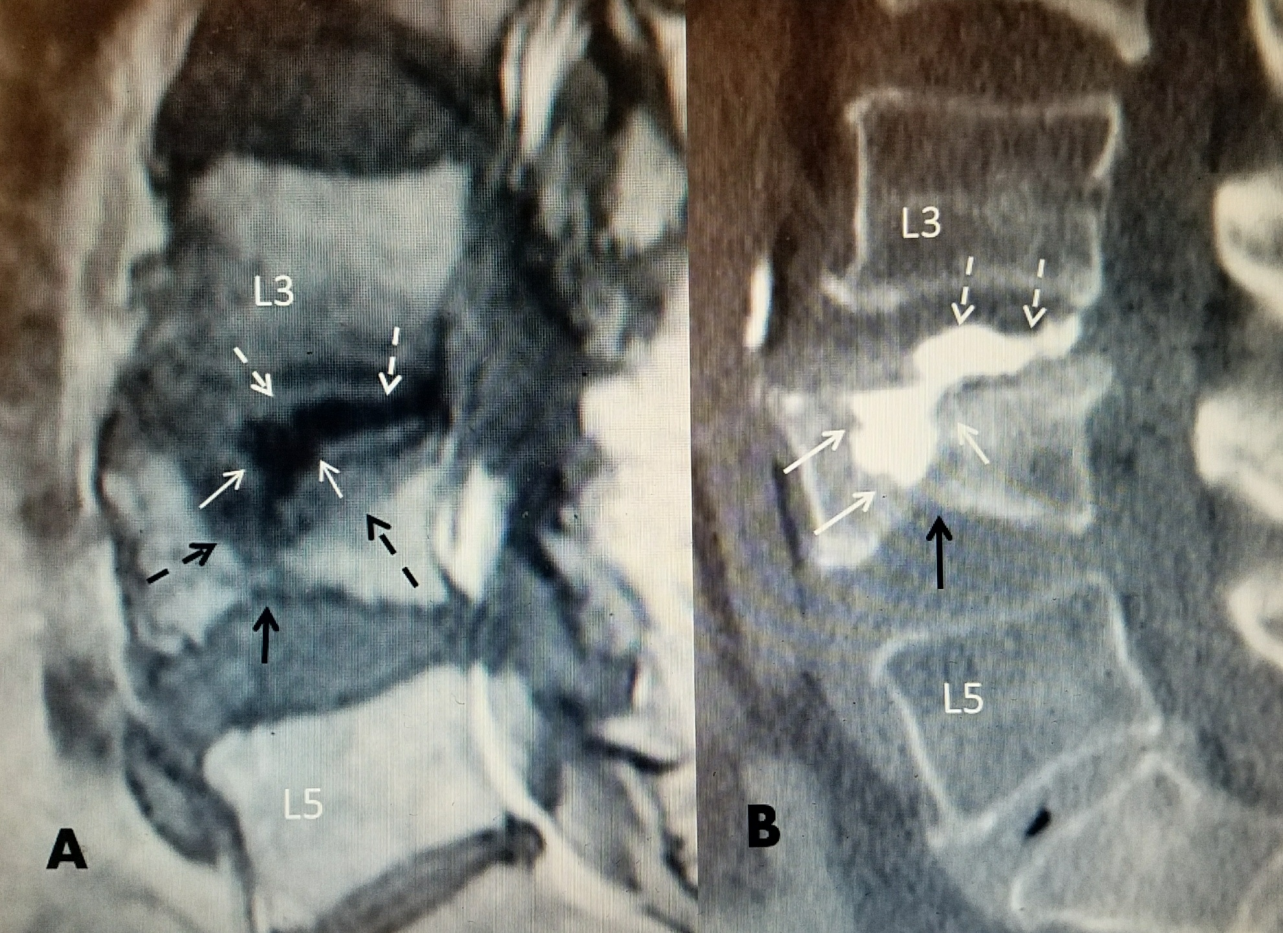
L4 wedge superior endplate fracture where cement inadequately filled the fracture A: T1 sagittal MRI post-L4 vertebral augmentation with polymethylmethacrylate (PMMA). There is only a partial fill of the large mid-vertebrae edematous fracture (dotted black arrows) with significant cement leakage into the middle and posterior L3-4 disc space (dashed white arrows). There is also an inferior endplate fracture (solid black arrow) creating biconcave vertebrae. B: Sagittal CT scan post repeat vertebroplasty. An extrapedicular approach was used to access the fracture site. Post repeat vertebral augmentation with Cortoss® at L4 showing an almost complete fill of the large midline fracture (solid white arrows). The small inferior endplate fracture is unchanged (solid black arrow). The previous leak of cement into the L3-4 disc space is unchanged (dashed white arrows).
